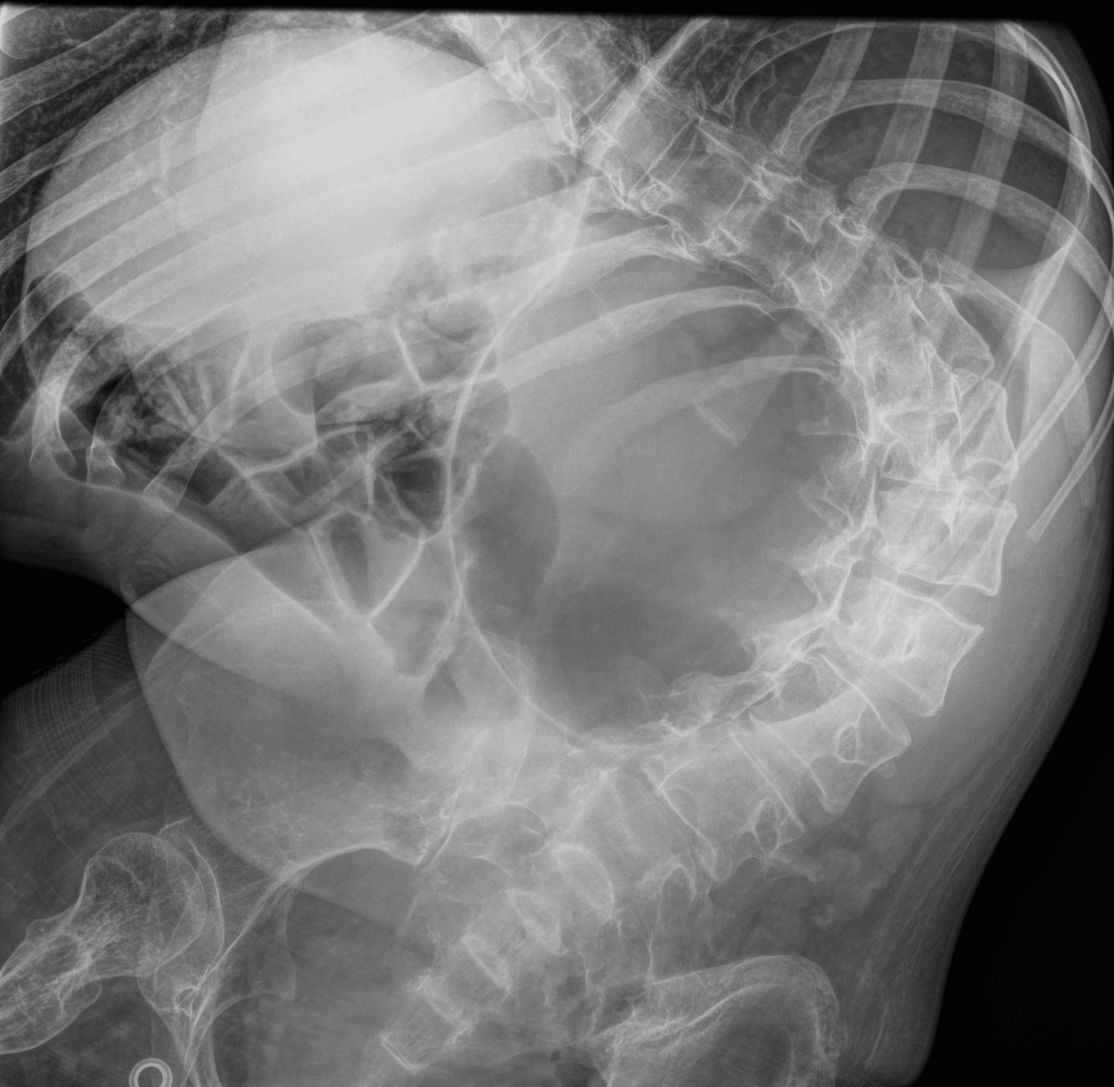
A simple abdominal X-ray shows significant abdominal distension.
